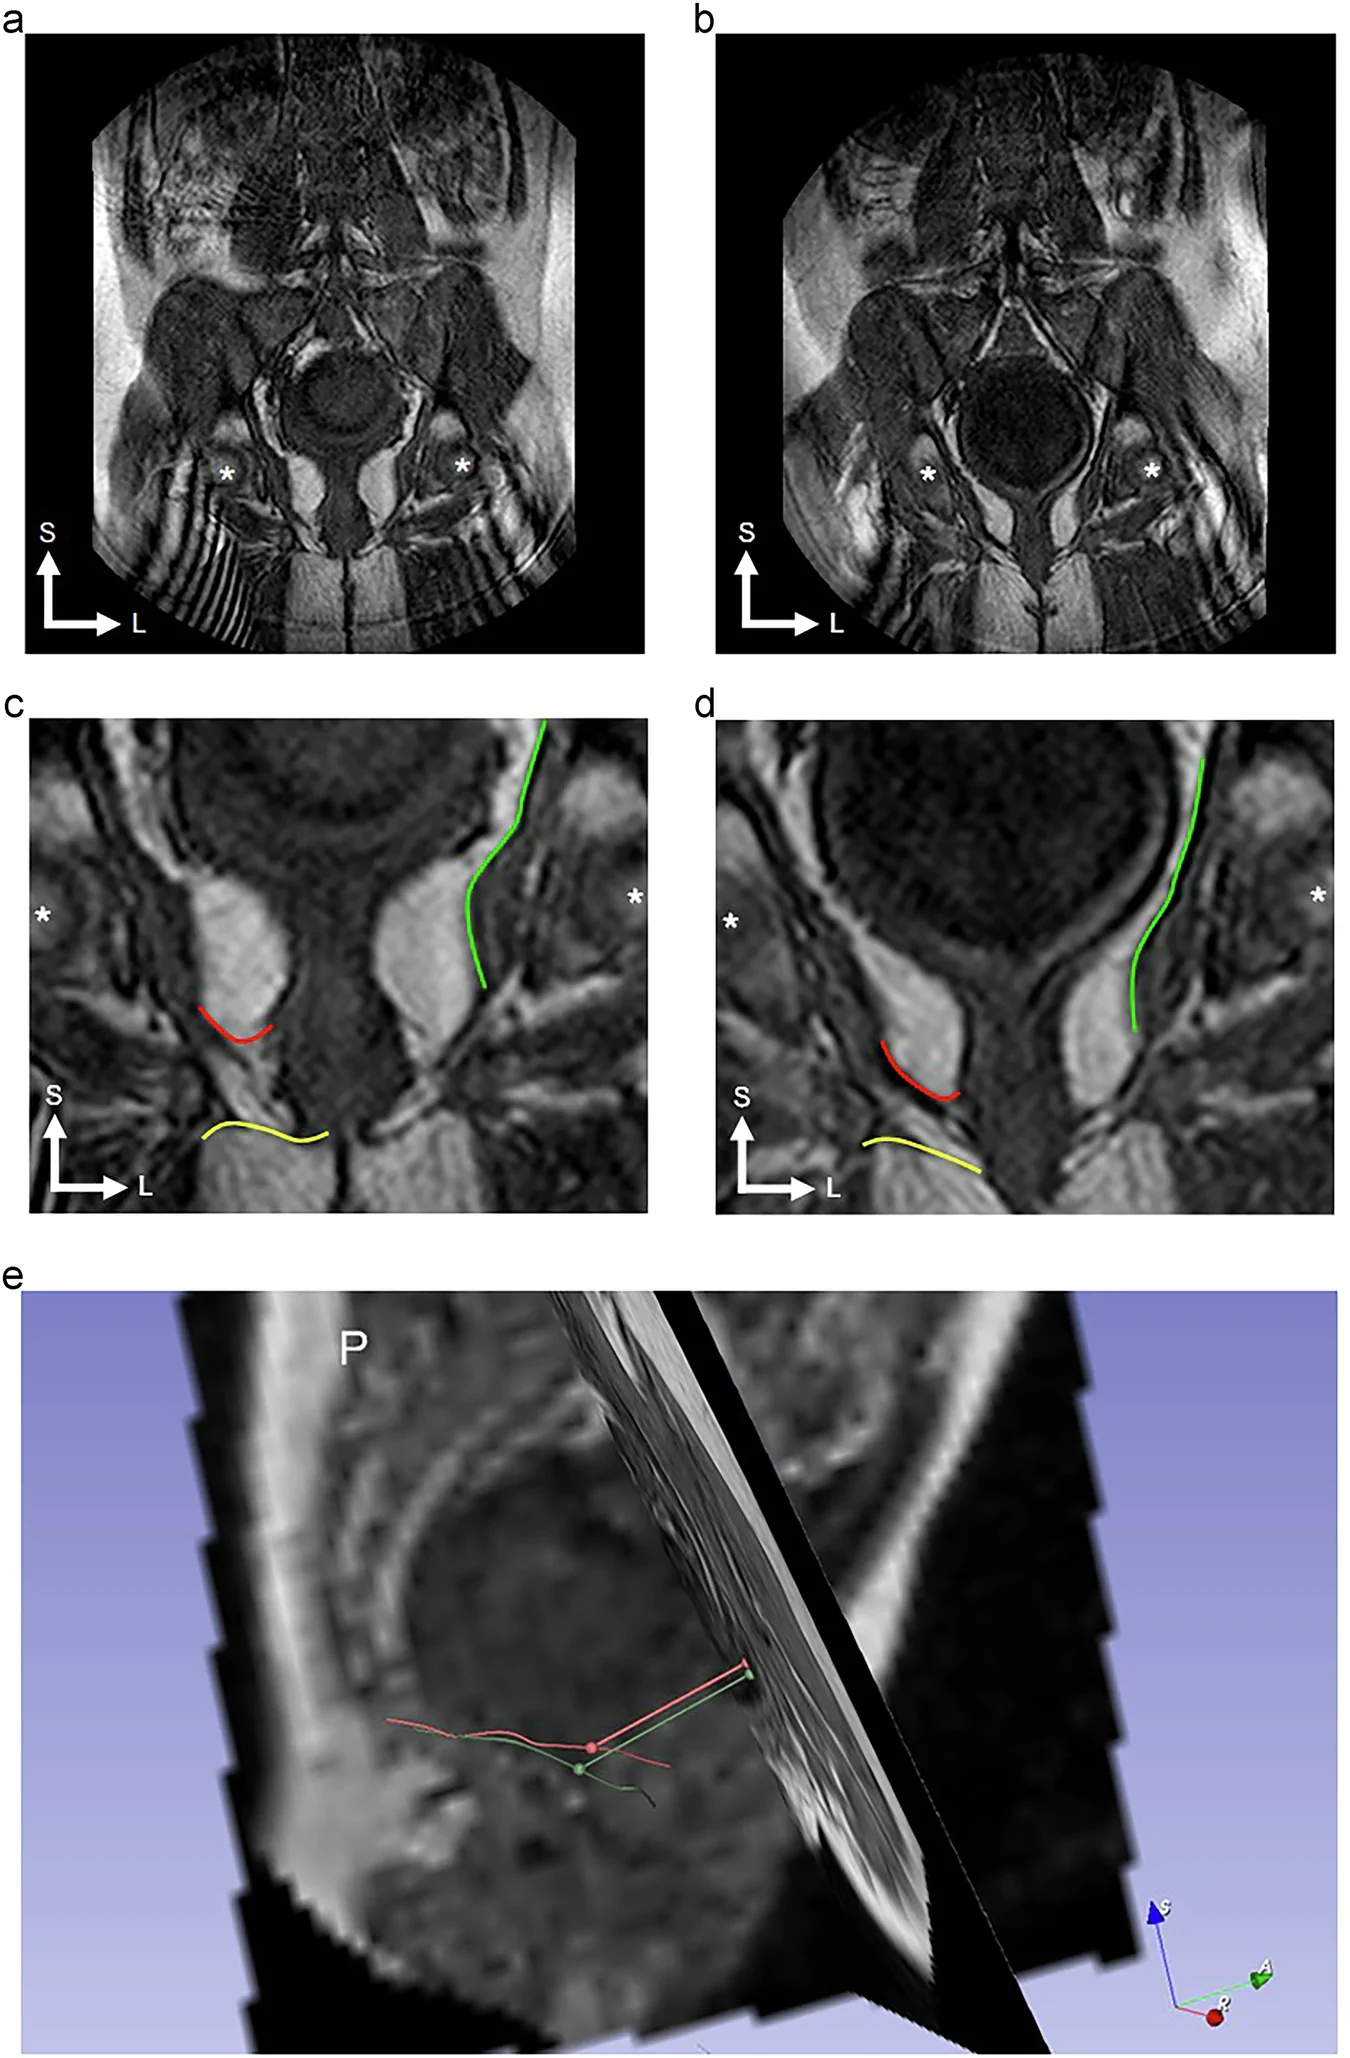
MRI of the pelvis in the coronal plane before entering labor (a, c) and during the SSL (b, d). The OIM (green line) was pushed externally by the descending fetal head. The superficial (yellow line) and deep (red line) transverse muscles were also flattened, compressed together, and shifted inferiorly, as indicated by the change in distance from the femoral head (*). A 3D view comparing the TALA before (red 3D line) and during (green 3D line) the SSL (e) based on image registration. The orthogonal distance between the TALAs and the upper brim plane decreases during the SSL. 3D, Three-dimensional; MRI, Magnetic resonance imaging; SSL, Second stage of labor; TALA, Tendinous arch of levator ani
